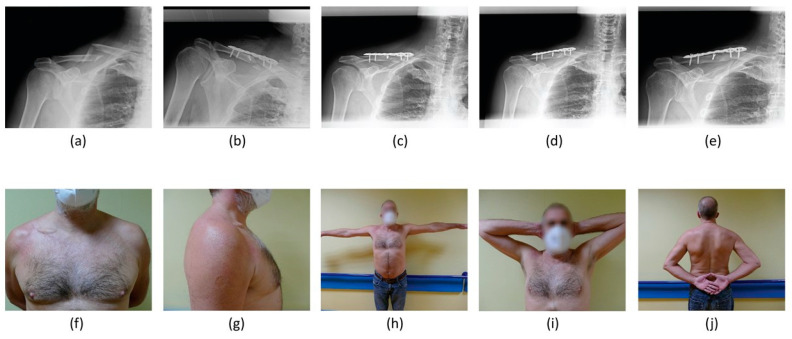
A 59-year-old male patient surgically treated for an AO B2 right ADMCF. Clavicle radiographic images at (a) the time of patient presentation in ER, (b) immediate post-operative radiographic control, (c) 30-day follow up, (d) 6-month follow up and (e) last follow up of 22 months. Last follow up clinical images showing (f,g) a mass of modest size on the right clavicular profile and patient’s satisfactory range of movement in abduction (h), extra-rotation (i) and intra-rotation (j).
